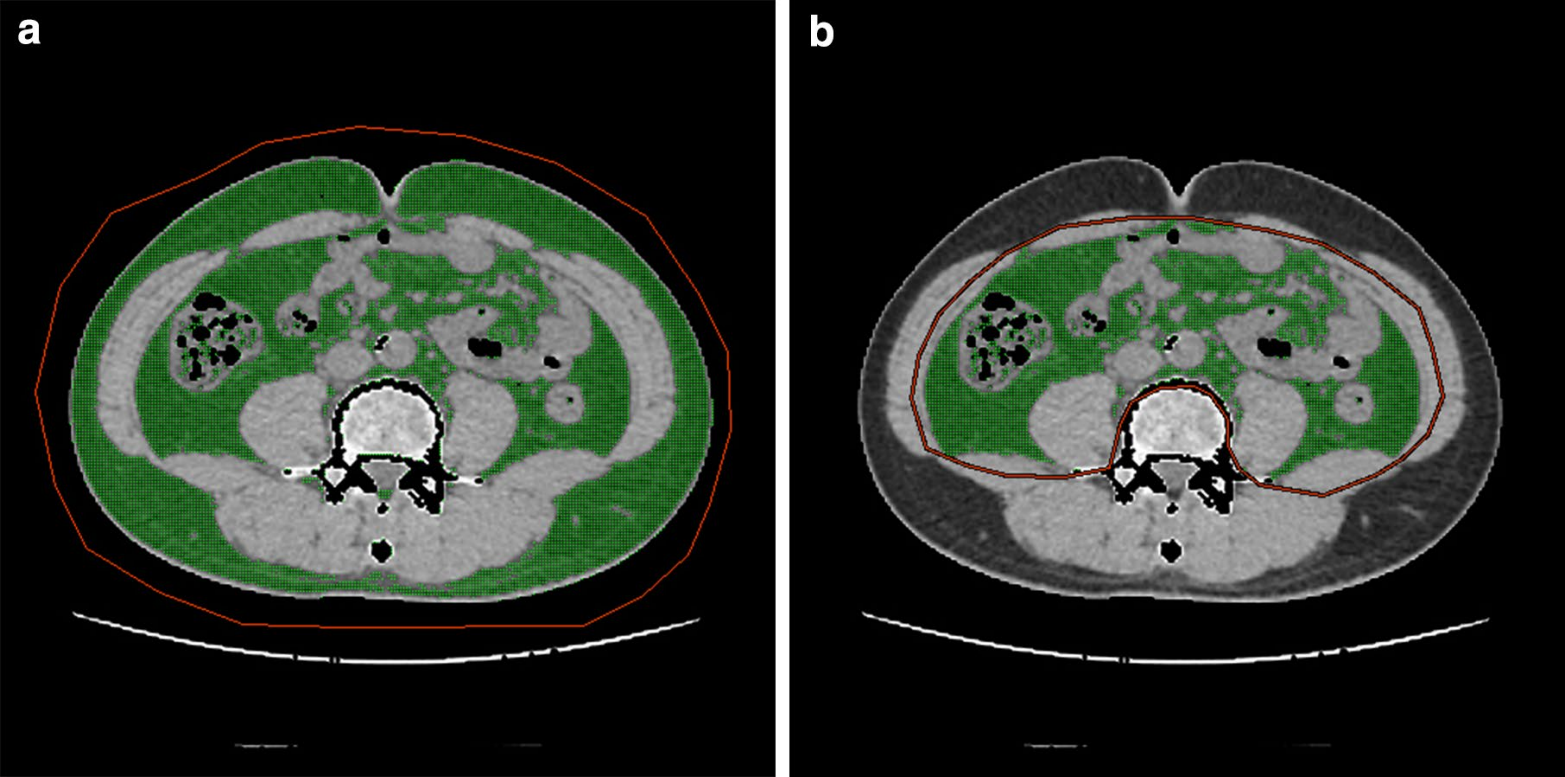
Graphics showing a method for determining the degree of fat distribution on computed tomography. a Area inside the *red line* is total fat area (TFA). b Area inside the *red line* is visceral fat area (VFA).
